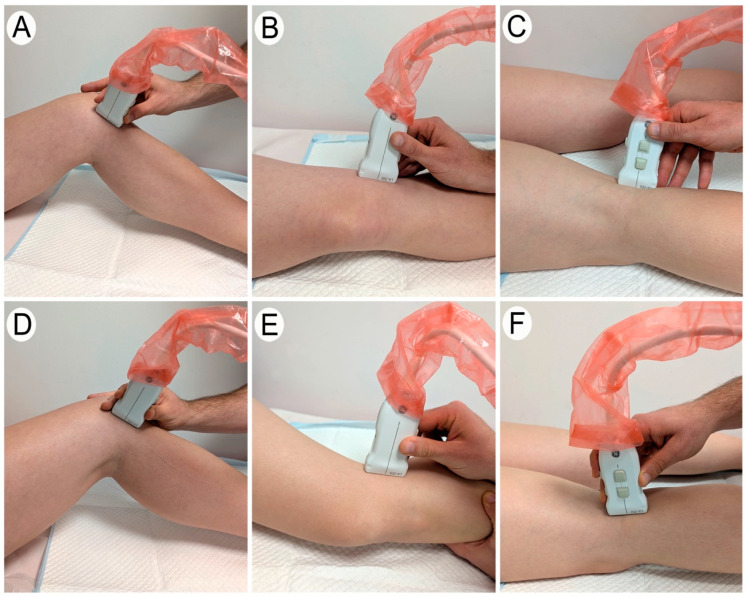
A transverse ultrasound scan of the medial and lateral meniscus. The images show the appropriate transducer placement to obtain a transverse view of each meniscal region. (A–C) Transverse ultrasound imaging of the medial meniscus: the anterior (A), middle (B), and posterior (C) regions. (D–F) Transverse ultrasound imaging of the lateral meniscus: the anterior (D), middle (E), and posterior (F) regions.
